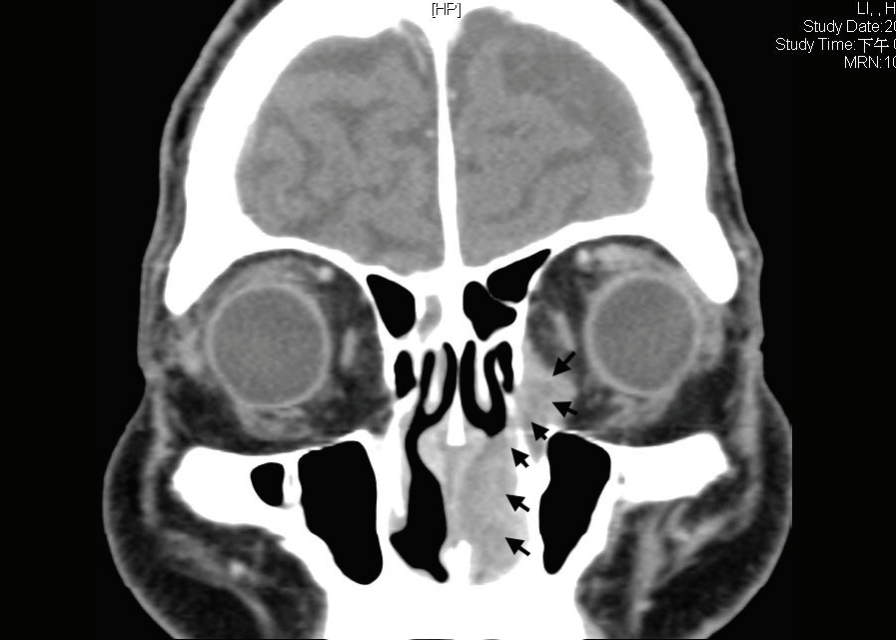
Contrast-enhanced CT demonstrated a dumbbell-shaped tumor in left medial canthal region and nasal cavity, connected by the nasolacrimal duct (arrowheads).
